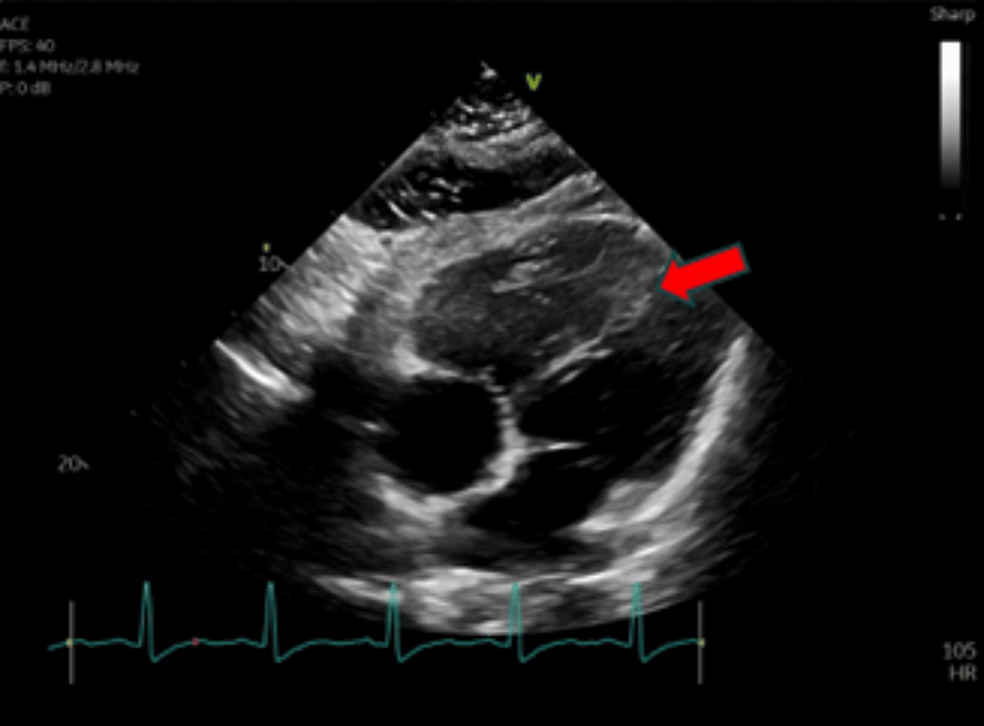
Transthoracic echocardiogram showing interventricular septal flattening, concerning for elevated right-sided pressures (red arrow).
